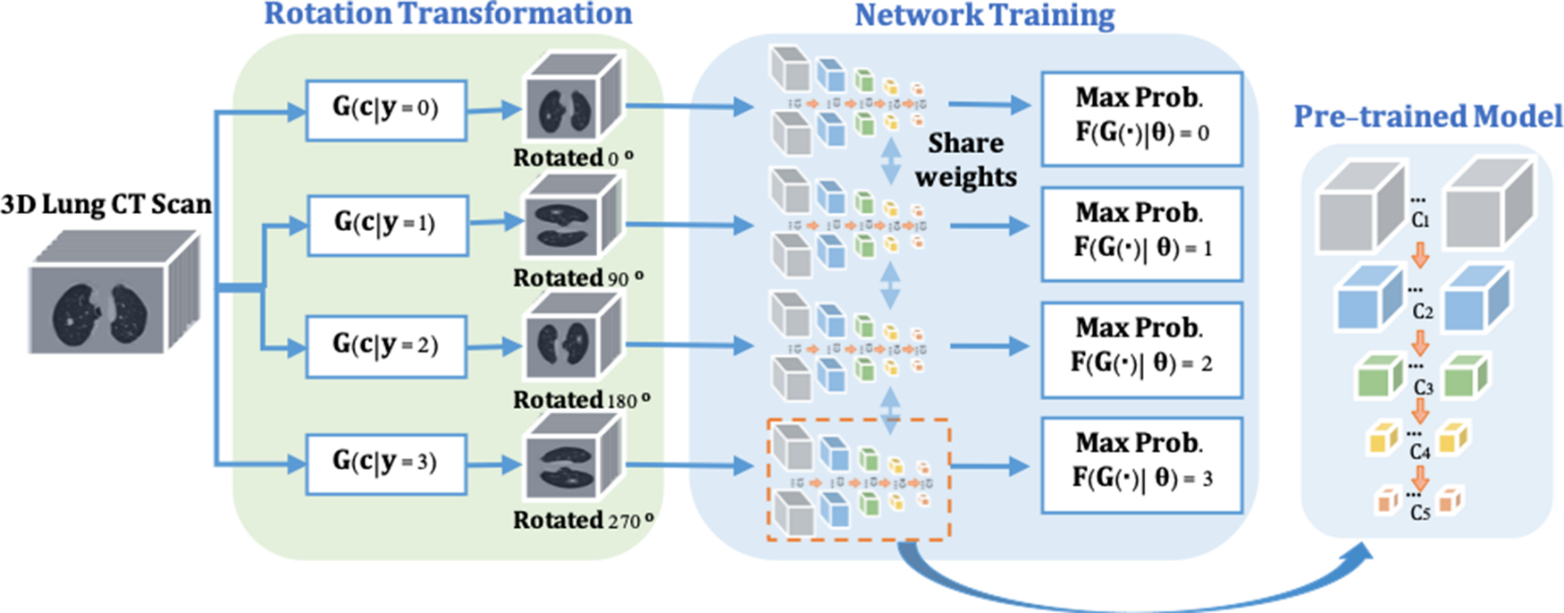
The pre-trained model training consists of two steps. (1) Rotate on input 3D CT scans with four angles as $0^{\circ },90^{\circ },180^{\circ },270^{\circ }$ by the rotation transformation network. (2) The rotation prediction pretext tasks uses the backbone network (*ResNet-18*) of the proposed nodule detector (*3DFPN*) for feature extraction and 2 fully connected (FC) layers to obtain the maximum rotation prediction probability.
